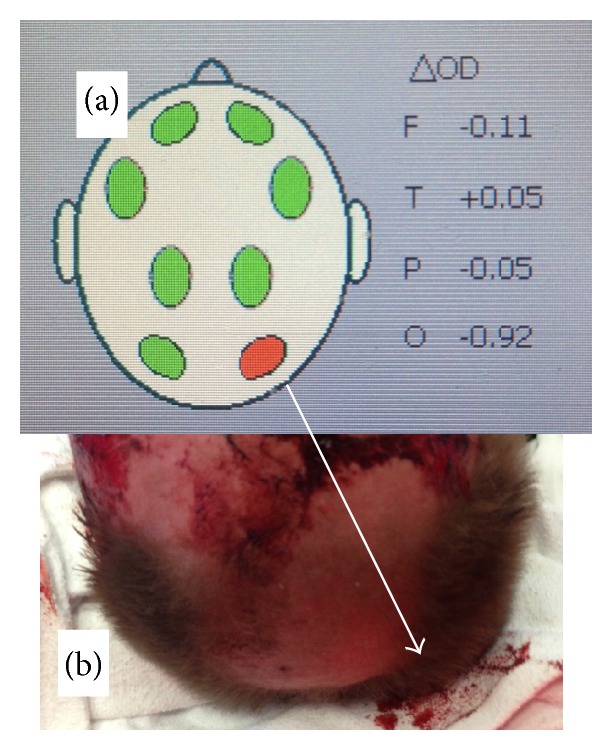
Photography (b) of a traffic victim's head (truck driver). Indicated (by white arrow) is a lacerated, occipital hematoma (as seen from cranial direction). This subcutaneous hematoma was part of the Infrascanner catchment volume, leading to the suggestion of an intracranial hematoma at this same spot ((a), Infrascanner screenshot). An intracranial hematoma was not confirmed by in-hospital cranial CT, stressing the need to meticulously circumvent subcutaneous hematomas at the measurement sites.
